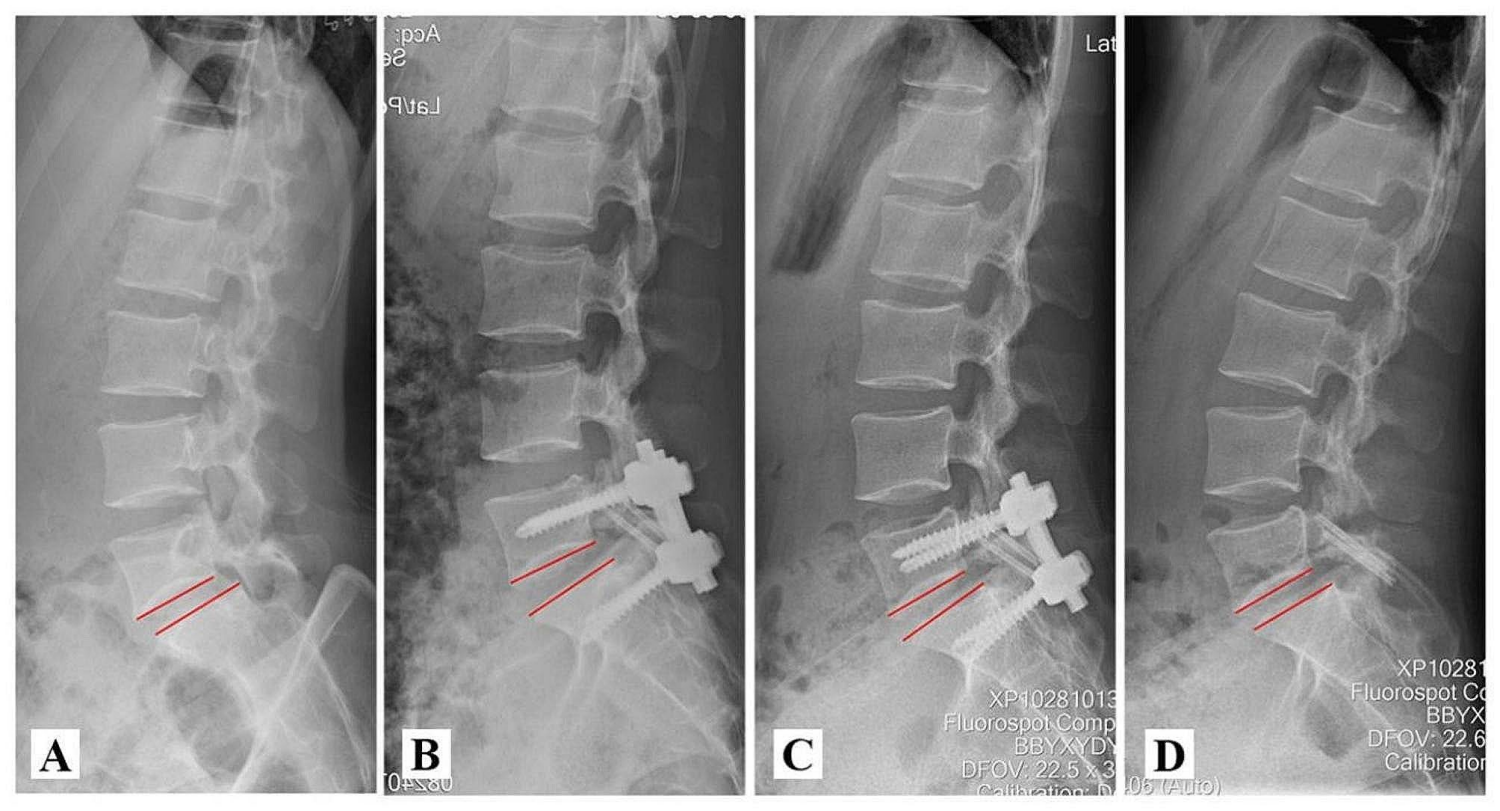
X-ray follow-up showed that intersegmental fixation could restore lumbosacral sagittal balance. (A) Preoperative. (B) Postoperative Day 3. (C) 6 months after surgery. (D) One year after surgery, the internal fixation was removed
